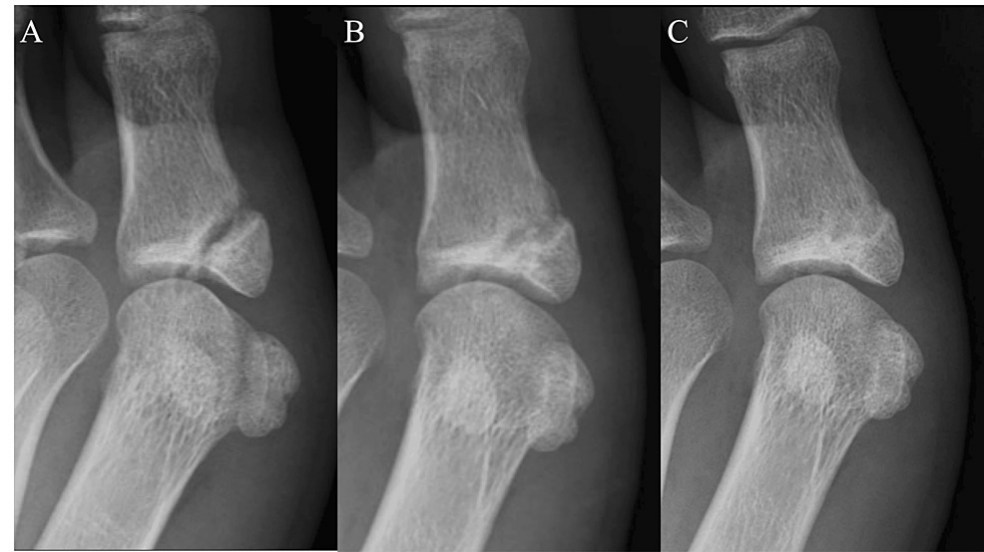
Radiographic findings of the left proximal phalanx of the great toe A: At the start of ESWT and PRP injection. B: Four weeks after treatment. C: Six weeks after treatment ESWT: extracorporeal shock wave therapy; PRP: platelet-rich plasma
